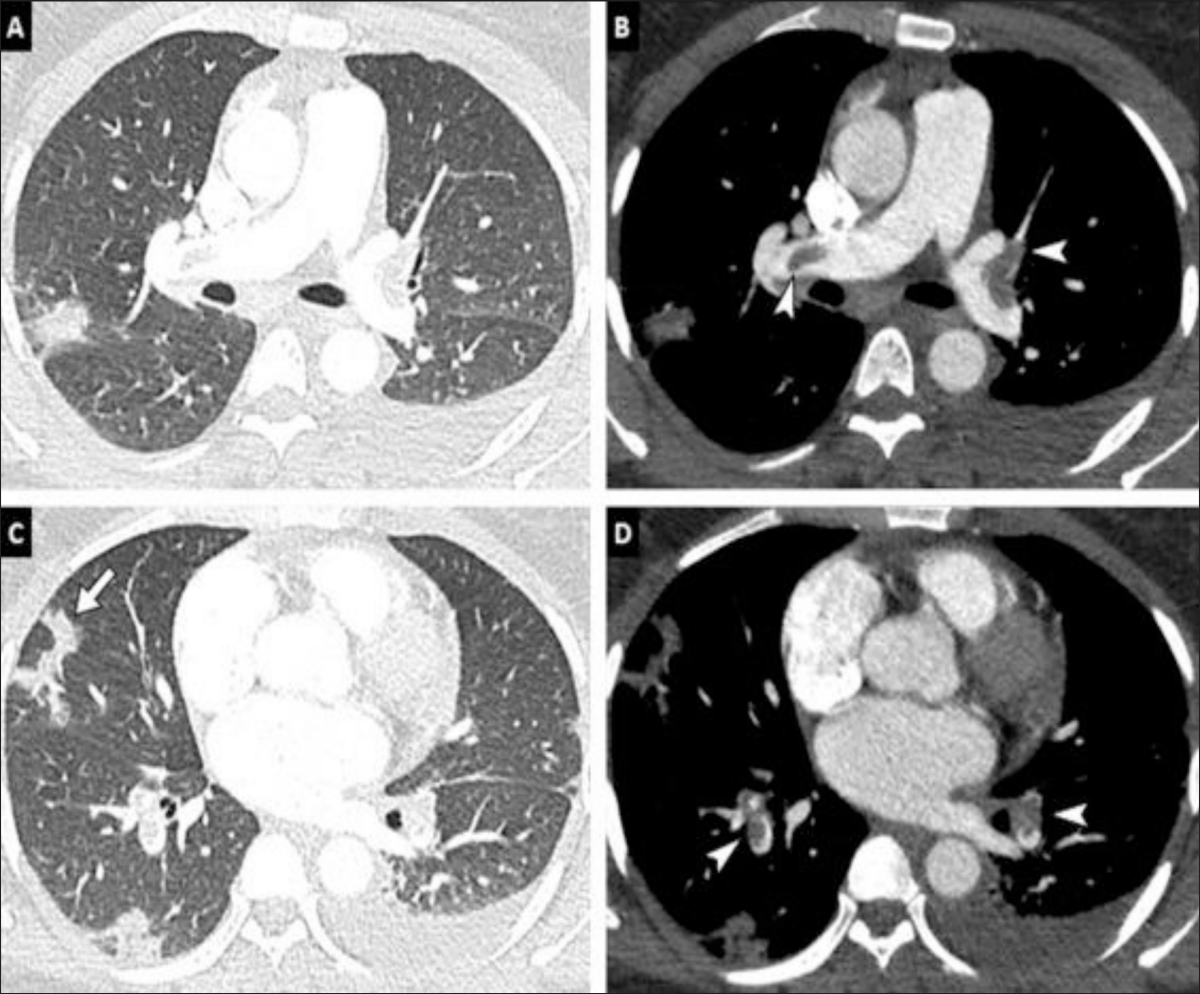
Chest CT-angiography of a 38-year-old patient with no pathological history with a positive PCR for COVID-19 pneumonia. Parenchymal window (A, C) shows peripheral foci of condensation. Mediastinal window (B, D) finds a bilateral proximal pulmonary embolism (endoluminal defects at the bilateral lobar level) as well as a left pleural effusion.
